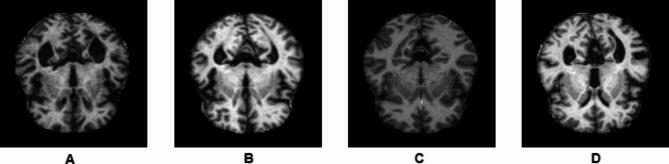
Sample MRI images of the brain from the first dataset, used to test our proposed model for identifying and classifying Alzheimer’s disease (AD). The MRI images include: (A) mild demented, (B) moderate demented, (C) non-demented, and (D) very mild demented.
